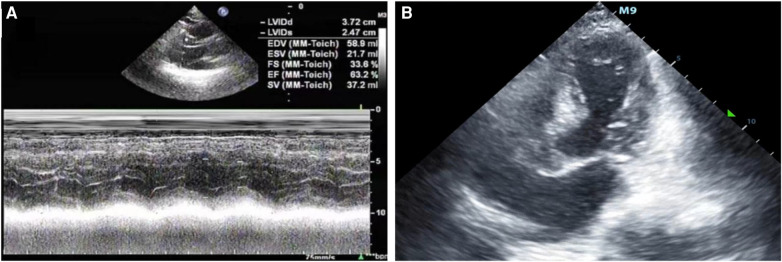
(A) The patient was admitted to the hospital with a left ventricular ejection fraction of 63.2% and normal systolic and diastolic function. (B) After admission to the ICU, bedside color ultrasound examination of the heart showed that the apex of the heart was round and blunt, with left ventricular systolic dysfunction and basal ganglia contraction.
